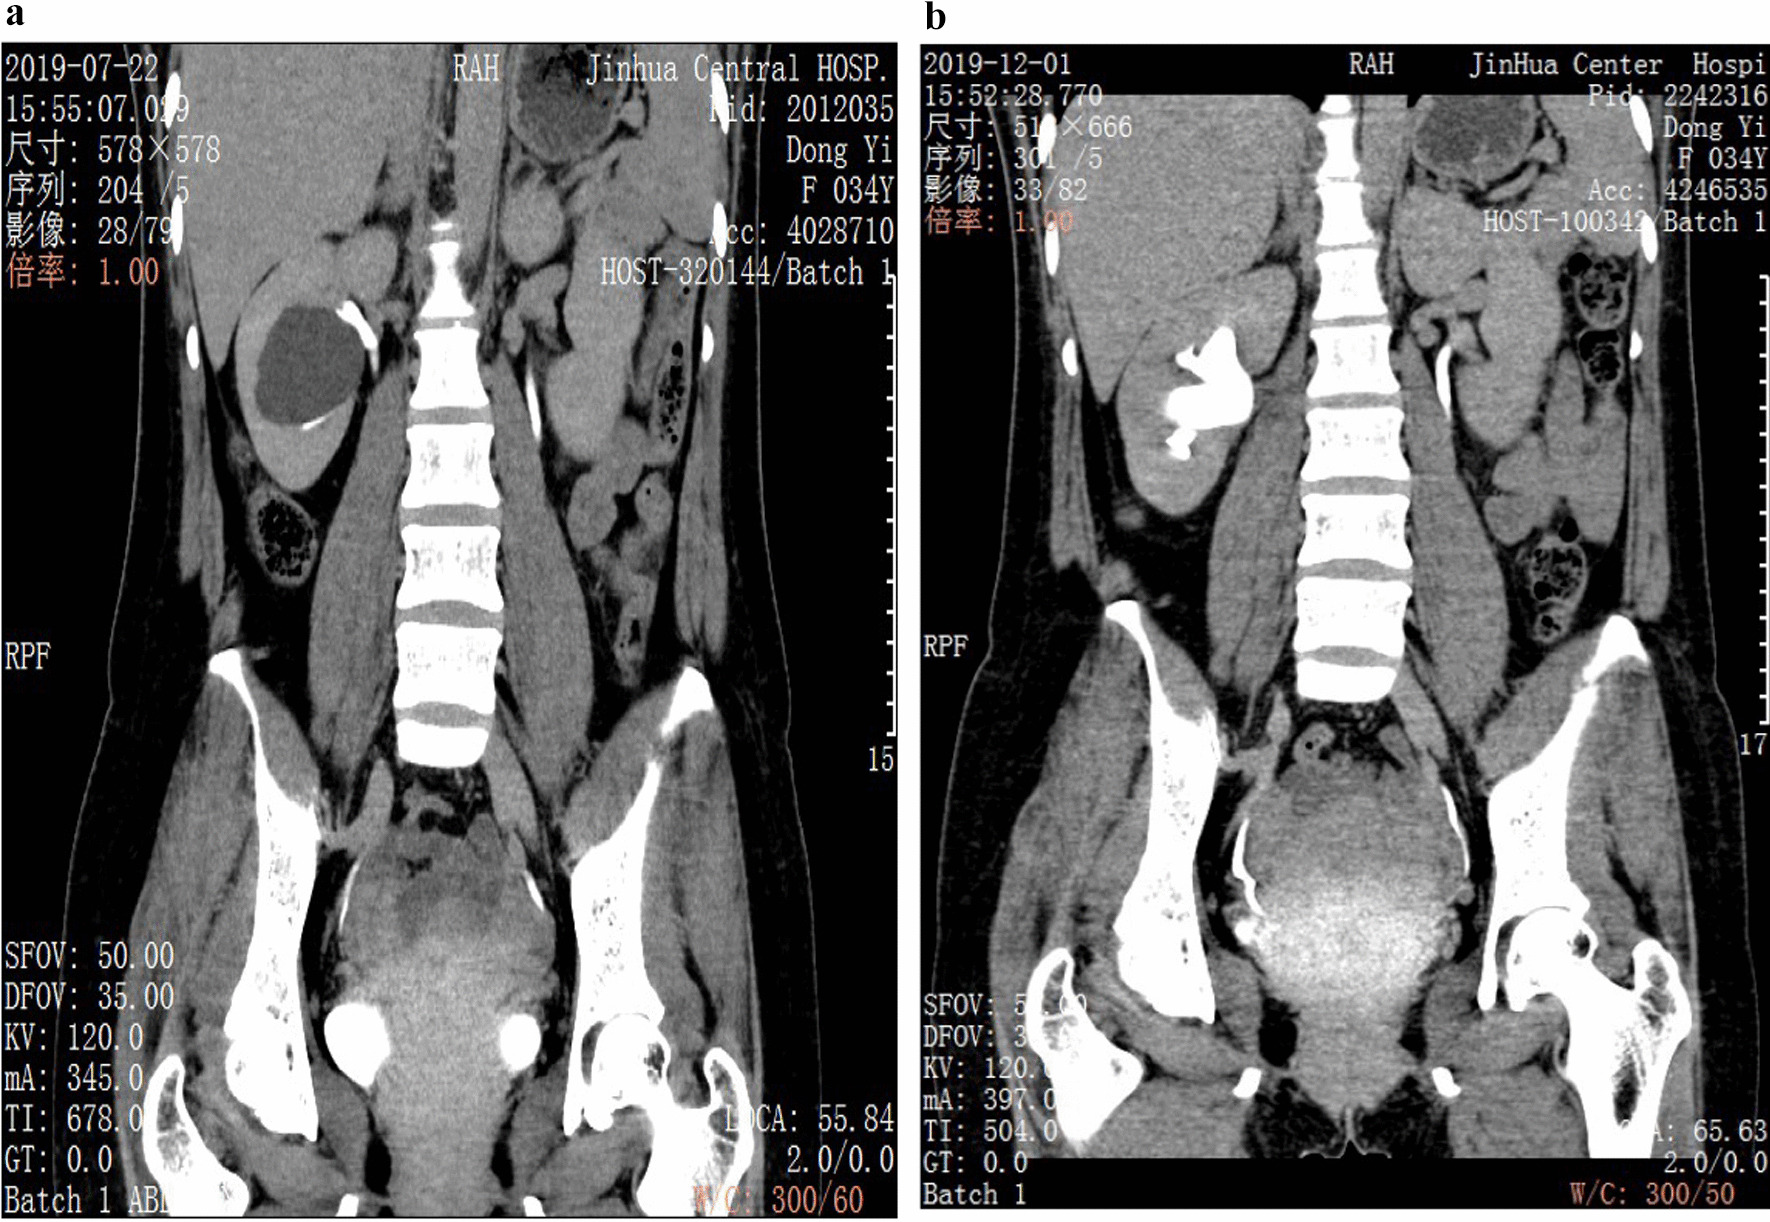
a The parapelvic cyst was shown in excretory phase imaging of preopretive urinary CT. b CT reexamined 4 months after surgery, the parapelvic cyst disappeared
